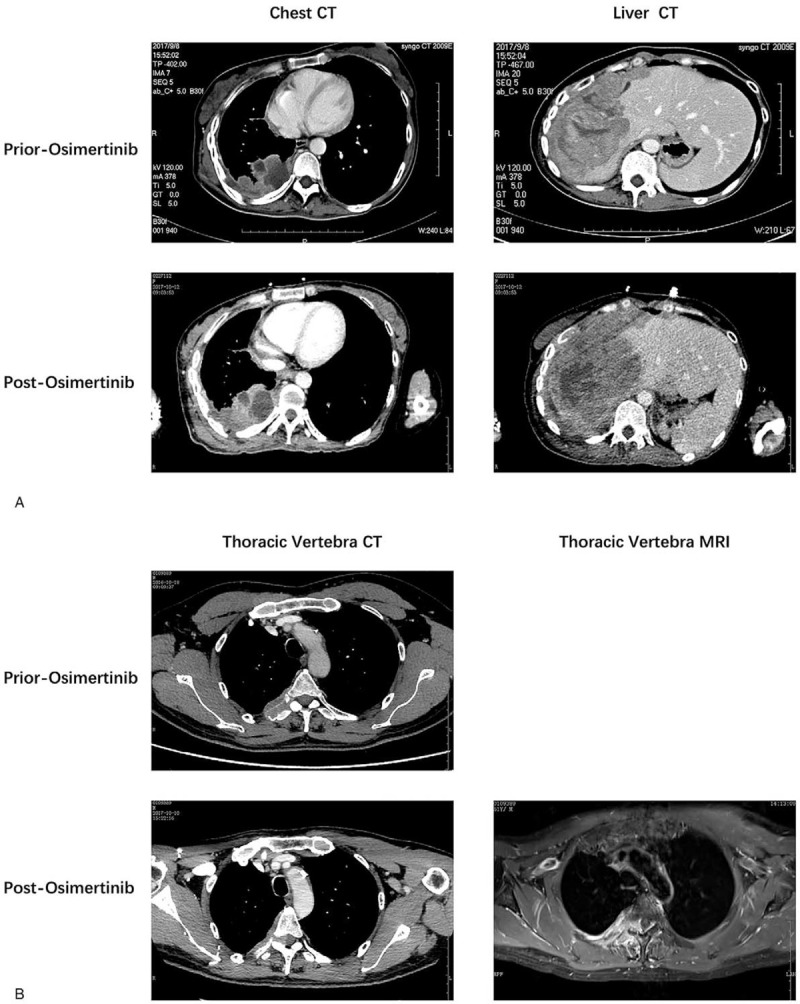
Computed tomography (CT) and magnetic resonance imaging (MRI) images of prior and postosimertinib treatment showed disease progress. (A) Case 1. (B) Case 2.
